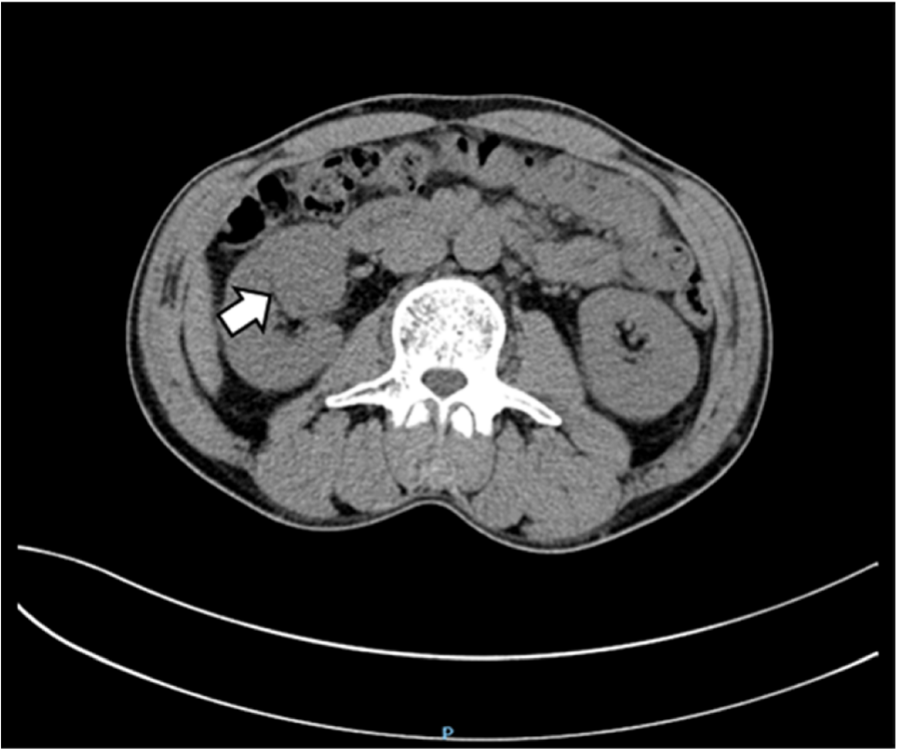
Plain computed tomography scan shows mass in the right kidney. Arrow: kidney tumor
